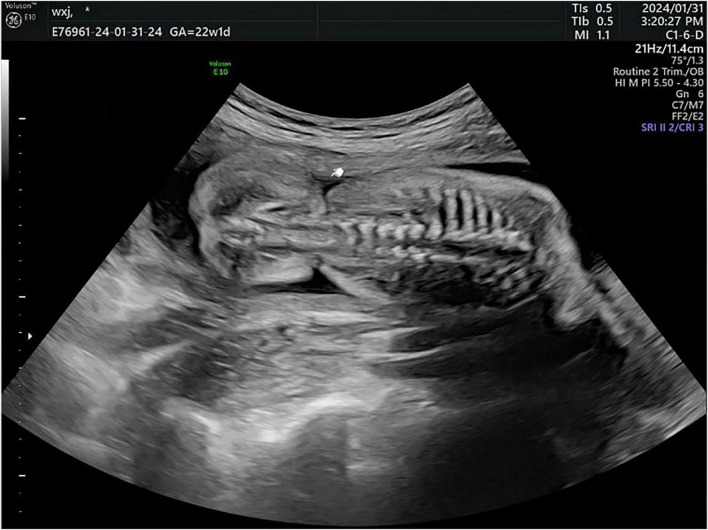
Ultrasonographic image of the fetal abdomen. The patient was a 35-year-old woman with twin-to-twin transfusion syndrome (TTTS) following fetoscopic laser photocoagulation (FLP). At 22 + 5 weeks of gestation, ultrasonography revealed amniotic bands wrapped around the recipient twin, with severe constriction of the abdomen.
